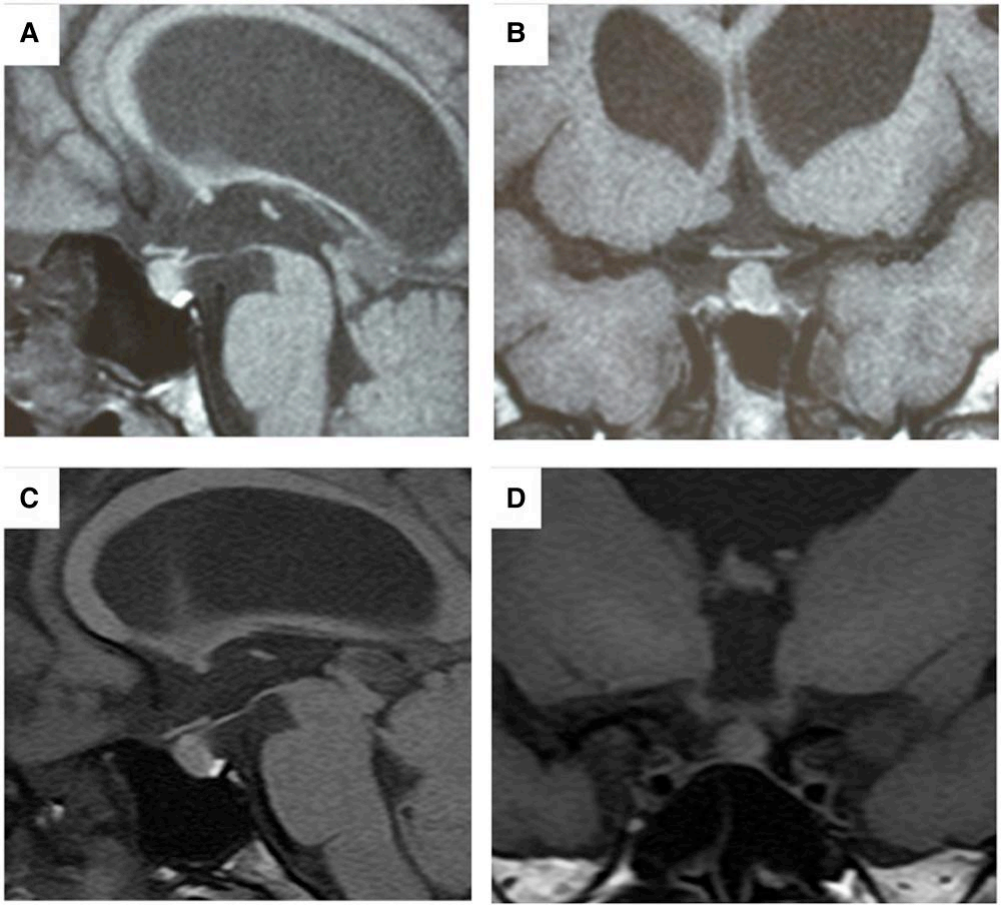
Brain magnetic resonance imaging (MRI) before radioiodine therapy. (A and B) before the final radioiodine therapy at 19 years of age; (C and D) after the final radioiodine therapy at 39 years of age. Sagittal (A and C) and coronal (B and D) images.
